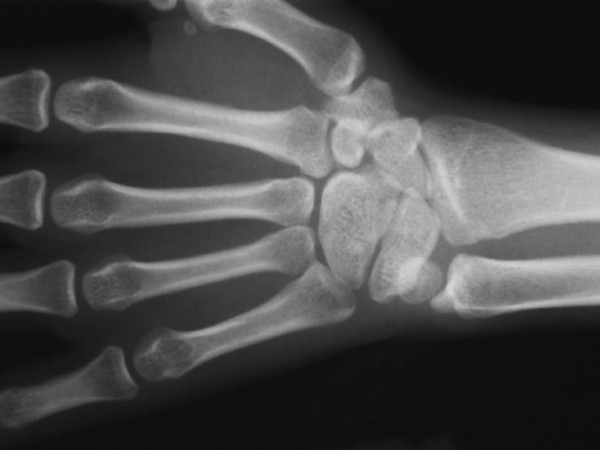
Hand x-ray showed synostosis between capitate-hamate-and lunate-triquetrum.
